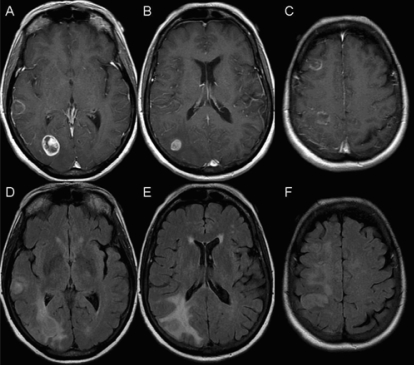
Magnetic resonance imaging slices showing glioblastoma multiforme (case 2). (A–C) Axial T1 post-gadolinium images and (D–F) corresponding axial flair (fluid-attenuated inversion recovery) slices at the same levels. Ring-enhancing masses can be seen in (A) the right temporal lobe, (A) right occipital lobe, (B) right inferior parietal lobe, (C) right frontal lobe, and (C) right superior parietal lobe. Under flair, hyperintensity can be seen around each lesion (D–F). Additional flair hyperintensity is seen intervening between the right frontal lobe and the right superior parietal lobe lesions (F).
